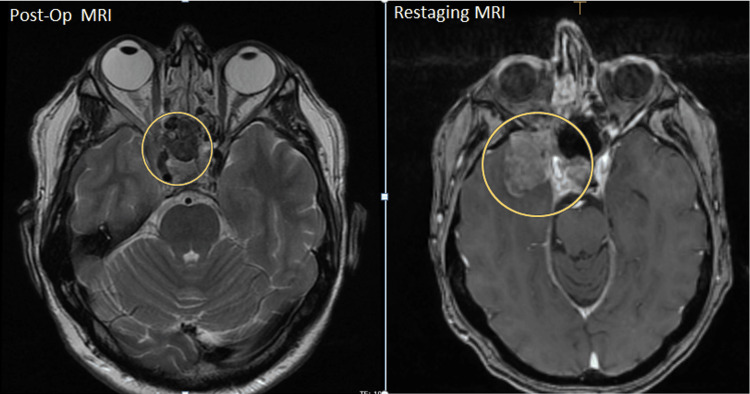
Re-staging MRI compared with postoperative imaging.
